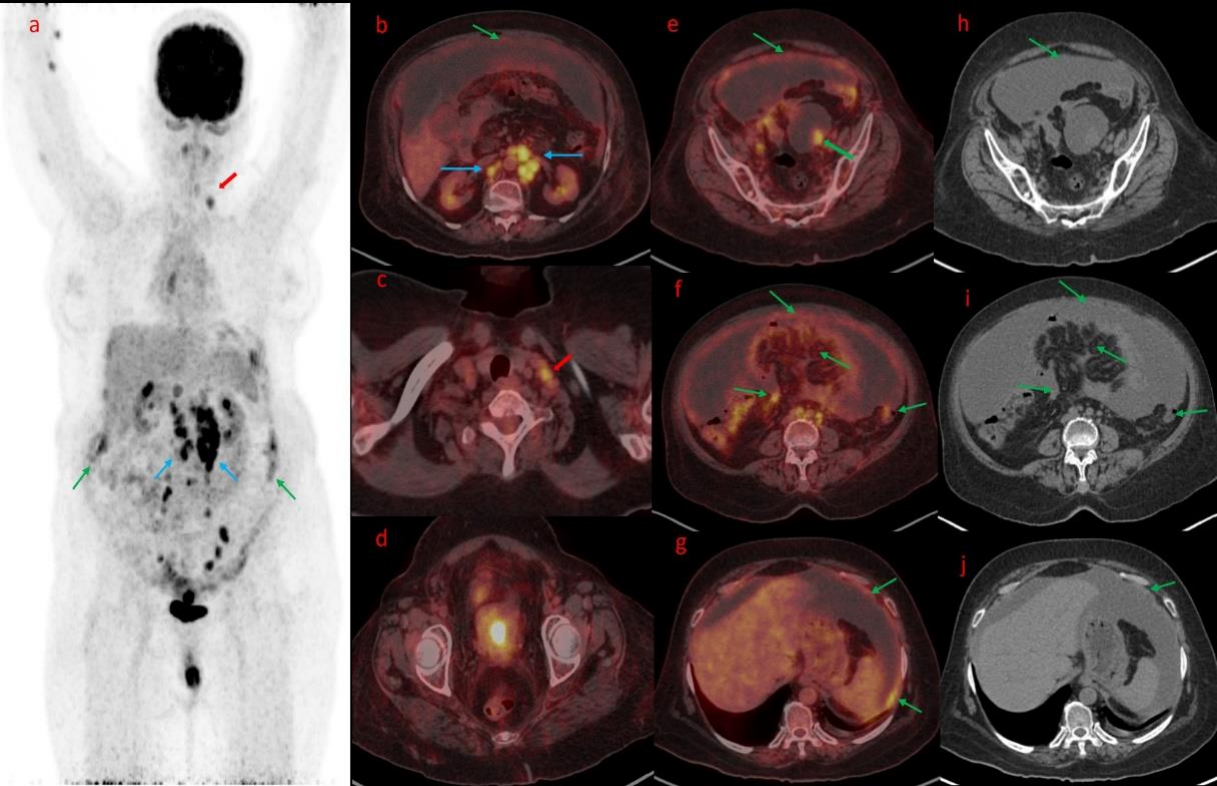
Maximum Intensity Projection image (a) revealed increased FDG uptake in the peritoneum (Green arrow) with nodularity and multiple FDG avid abdominal nodes (blue arrow) and a left supraclavicular lymph node (Red arrow). Axial section Fused FDG PET/CT (b, c) revealed gross ascites with FDG avid peritoneal thickening (green arrow), Multiple FDG avid abdominal nodes (blue arrow), and left supraclavicular lymph node (red arrow). Axial Fused PET/CT (d) from the pelvis revealed increased FDG uptake in the endometrium cavity. Axial Fused FDG PET/CT images (e, f, g) and corresponding CT (h, i, j) revealed diffuse peritoneal thickening with peritoneal and serosal deposits, omental thickening with increased FDG uptake (green arrow), and ascites. Fused Axial PET/CT (e) and corresponding CT images (h) revealed a solid cystic lesion in the bilateral adnexal region with increased FDG uptake in the solid component of the left adnexa (e, green arrow)
